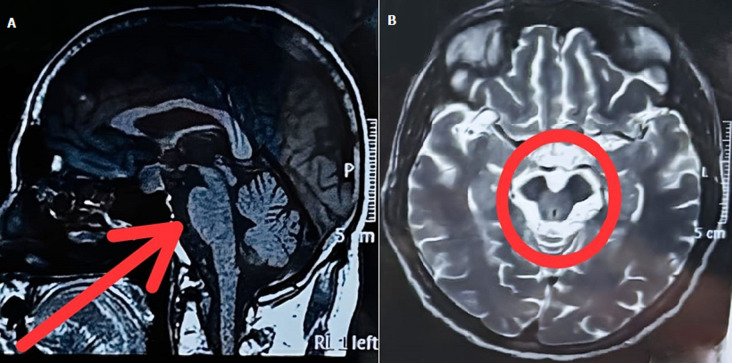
A, B) sagittal T1-weighted (red arrow) and axial T2-weighted (red circle)
